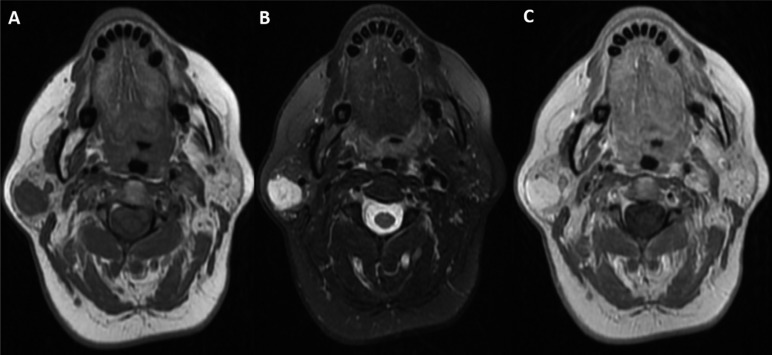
A) MRI T1 reveals in the right superficial lobe of the parotid gland a well defined, hipointense and lobulated intraparotid tumor of approximately 3cm. It is located posterior and lateral to the retromolar vein. Rest of the glandular parenchyma has normal morphologic features and intensity. B) MRI T2, shows the same image but the tumor is hyperintense. C) MRI T1 with contrast, shows the same image but with intense enhancement of the tumor.
